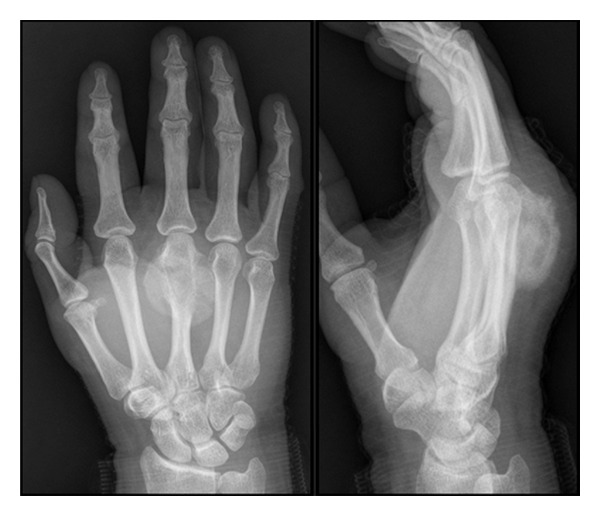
Radiograph taken preoperatively showing rapid growth of the dorsal mass.
